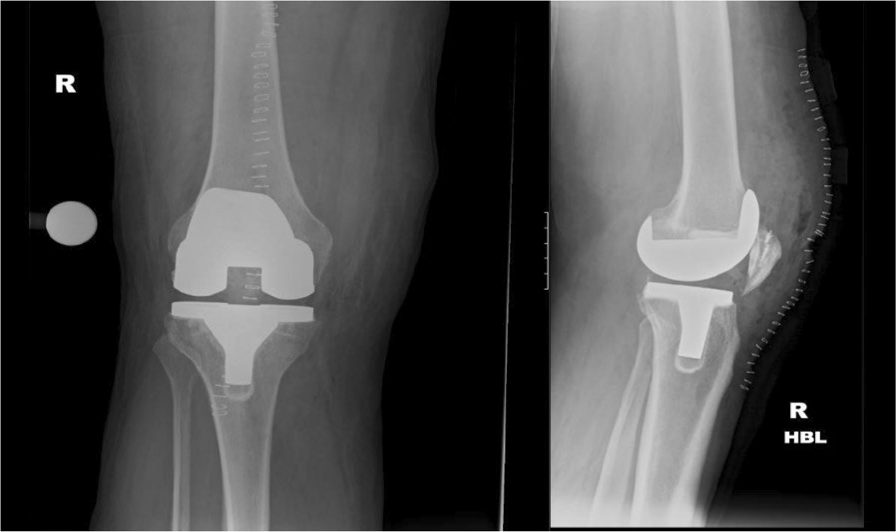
Showing immediate postoperative radiographs, which confirm adequate prosthetic implantation and positioning
